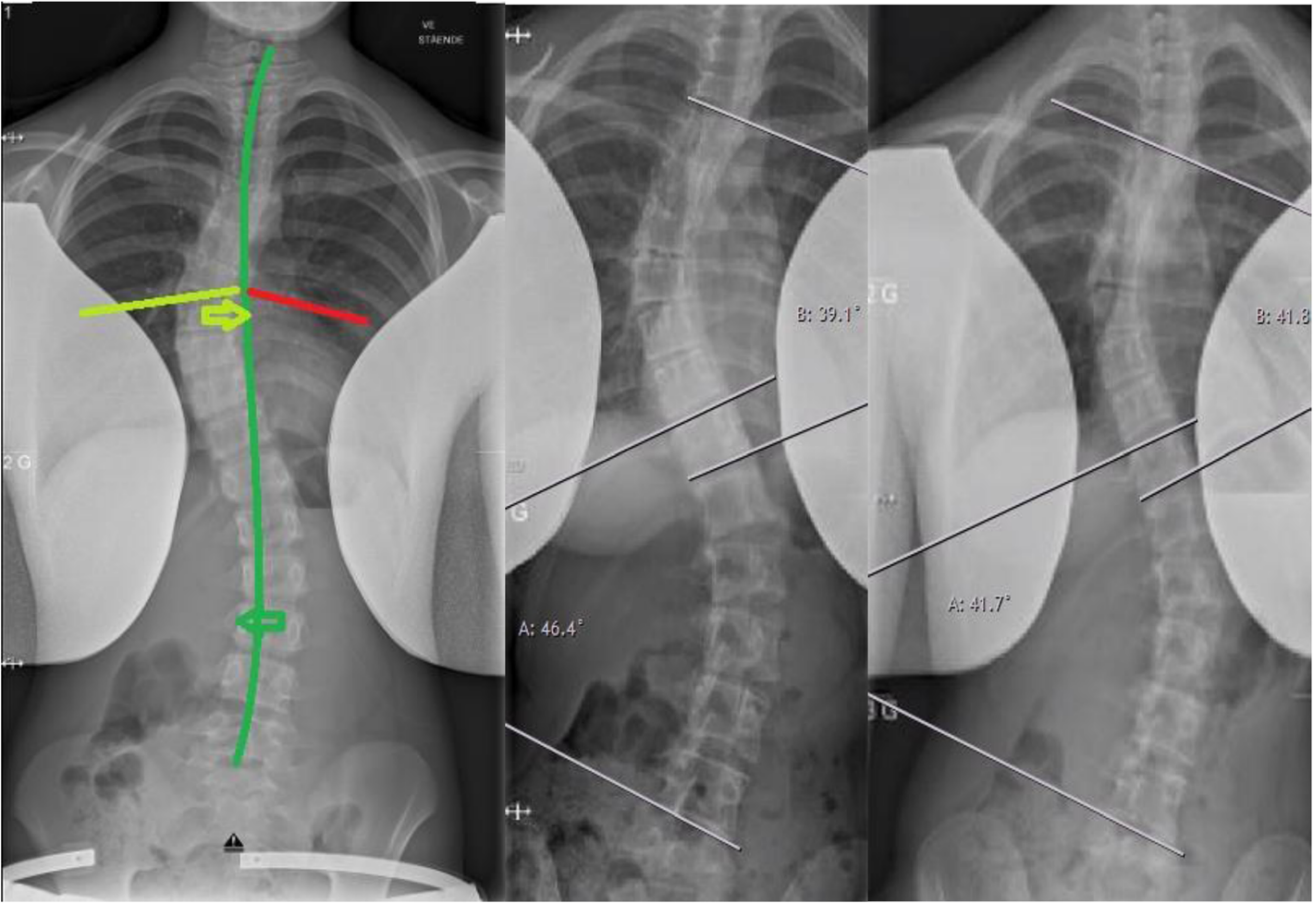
Changes in radiographic parameters. To the left: dark green, significant improvement in Cobb’s angle (curves) and Nash and Moe’s classification (error); light green, insignificant improvement in Nash and Moe’s classification (error) and in rib vertebra angle (line); red, insignificant deterioration in rib vertebra angle (line). To the middle and right: an example of pre- (middle) and post-injection (right) radiographs for patient number 9, where there is a smaller lumbar Cobb’s angle and larger thoracic Cobb’s angle after injection of botulinum toxin A
